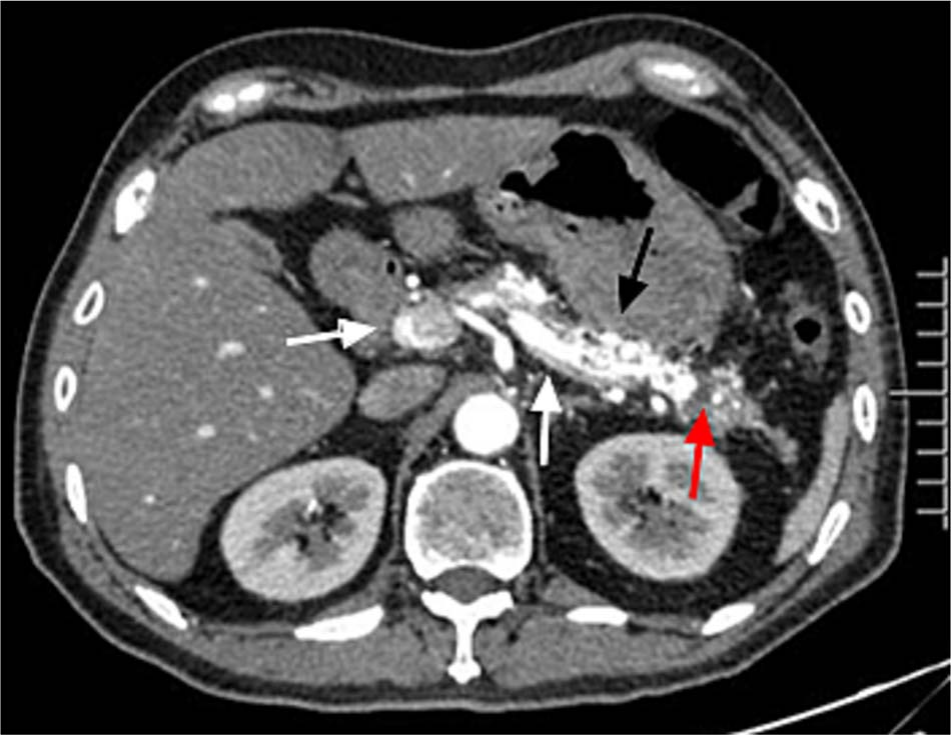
Enhanced CT showed tortuous dilated arteries in the pancreas body and tail (black arrow) with a small pseudocyst (red arrow) and early opacification of the splenic vein and portal vein (white arrows).
